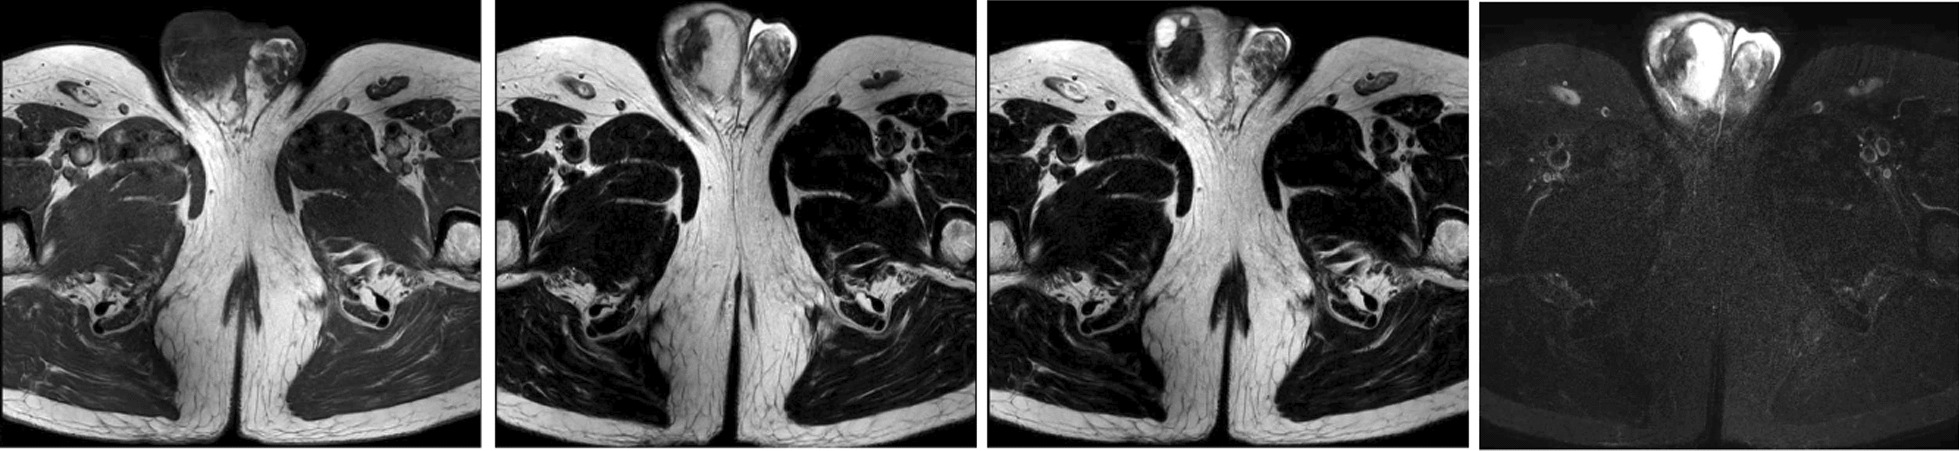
Preoperative magnetic resonance imaging (August 2021) showing mixed signals of cystic and solid nature in the right epididymis
